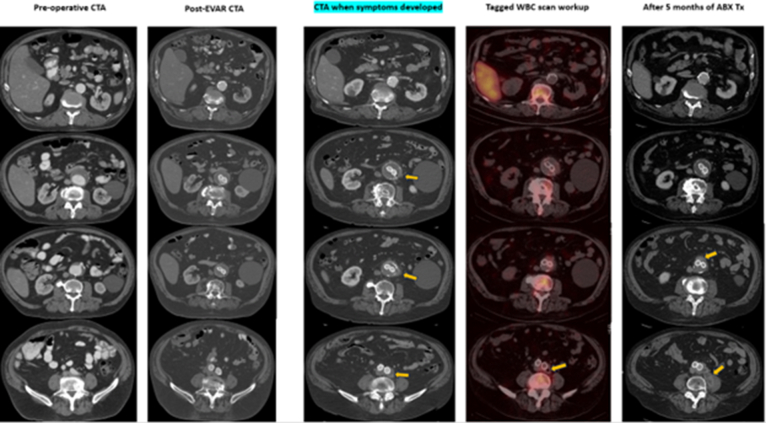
Captured timeline of the delayed development of *Coxiella burnetii* endograft infection after endovascular aortic repair (EVAR). *Arrows* marking retroperitoneal thickening, fat stranding, and enlarged lymph nodes next to the aorta at the time of initial symptoms. Tagged white blood cell (*WBC*) scan was negative. Antibiotic therapy (*ABX Tx*) was ineffective, and the infection continued to spread locally. *CTA*, Computed tomography angiography.
